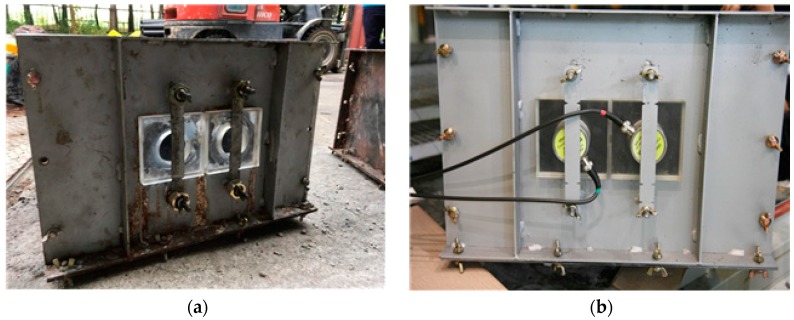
Preparation of the steel mold for surface wave velocity measurement: (a) mounting the acrylic panel within the steel mold before the installation of the ultrasonic transducer and (b) the steel mold after the installation of the ultrasonic transducer.
